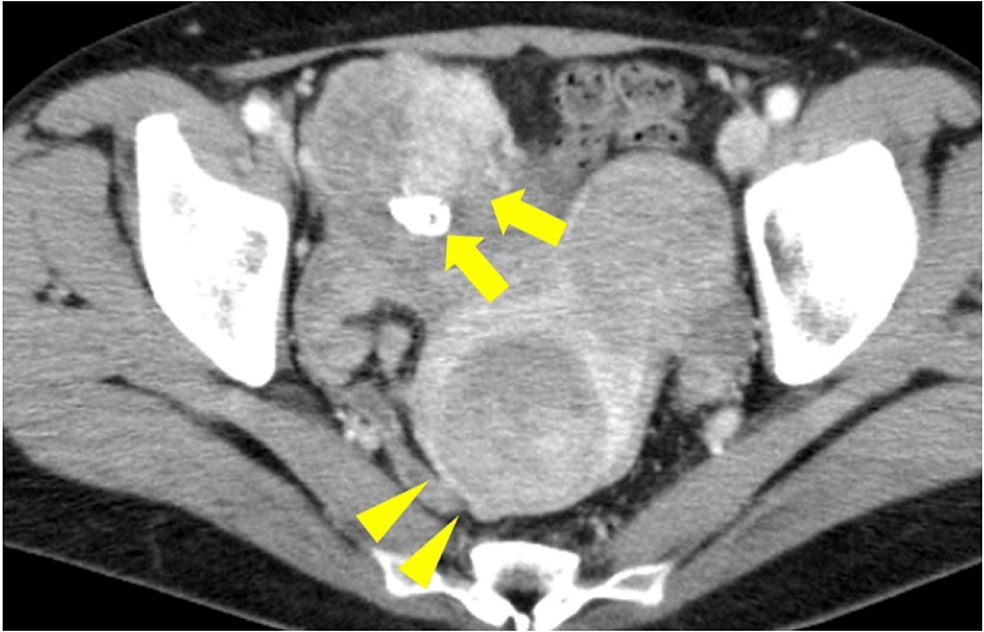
The preoperative abdominopelvic contrast CT image (axial view). A 50 x 68 mm mass lesion (arrow) with a heterogeneous contrast effect can be observed on the right side of the pelvis. The tumor had moved ventrally and grown in size compared to prior imaging (Figure 3). Arrowheads represent uterine myomas that have already been noted.
